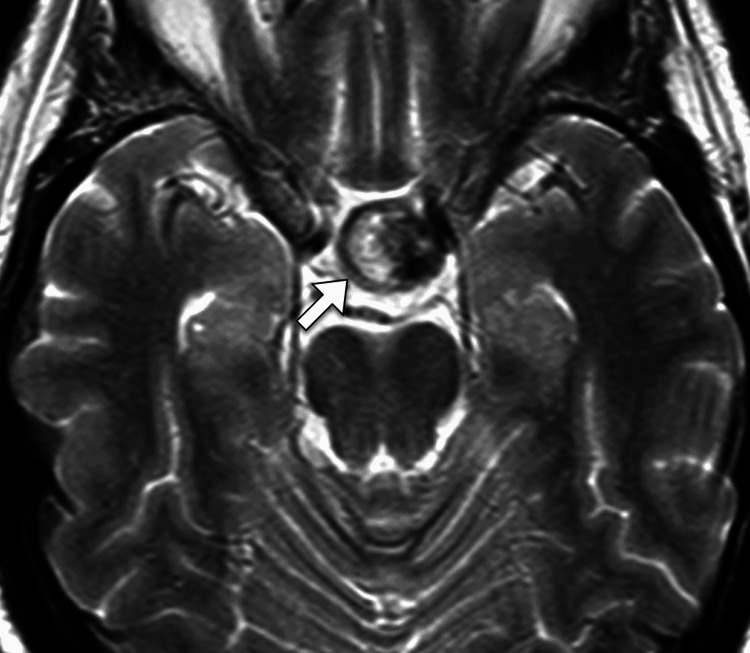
Axial T2-weighted magnetic resonance image of the brain demonstrating a mixed signal intensity sellar lesion with hypointense components (arrow)
